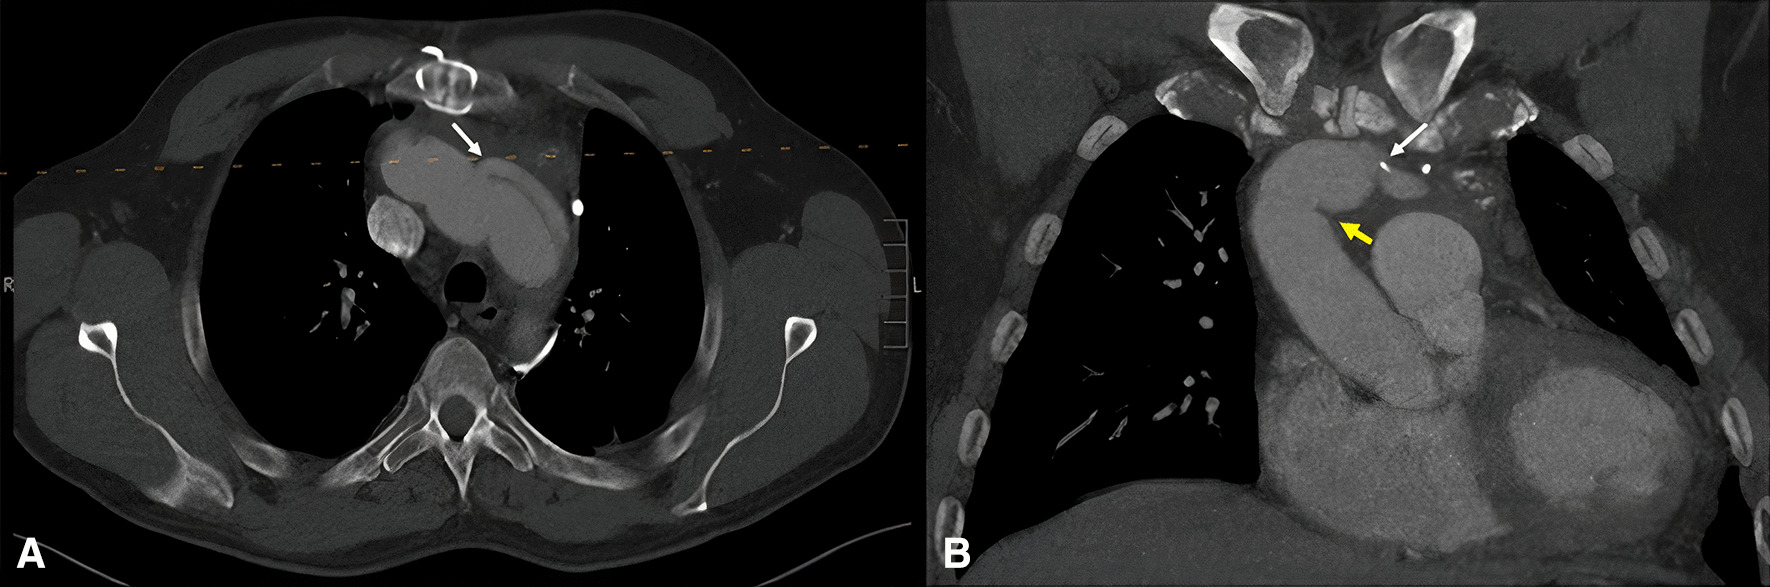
A Axial and B coronal CT angiography images showing the anastomosis site of the reimplanted left subclavian artery and the aortic arch (white arrow) as well as the anastomosis between the distal ascending aorta and the Dacron tube (yellow arrow)
